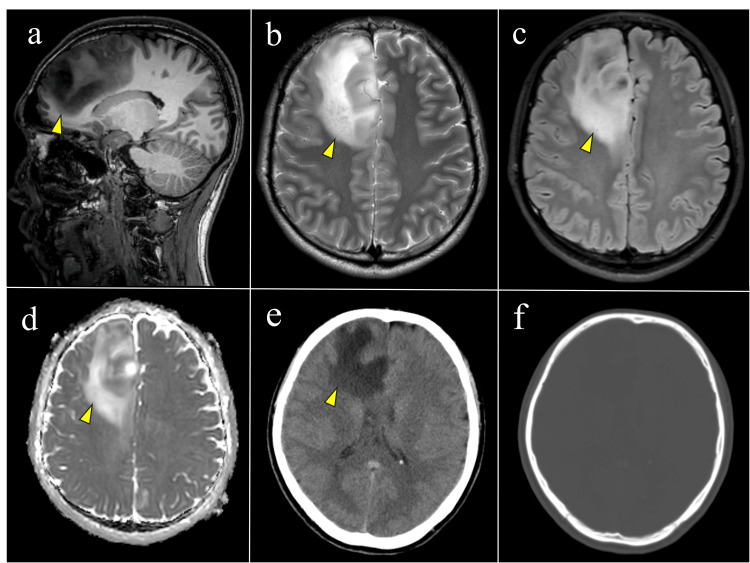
Brain MRI and CT findings (a) Sagittal T1-weighted image showing a hypointense lesion in the deep white matter of the right frontal lobe (yellow arrowhead). (b) Axial T2-weighted image and (c) axial FLAIR image showing a hyperintense lesion with an ill-defined margin. (d) ADC map showing no restricted diffusion in the same region. (e) Non-contrast CT showing a low-density area in the right frontal white matter (yellow arrowhead) without evidence of hemorrhage. (f) Bone-window CT confirming the absence of calcification. MRI, magnetic resonance imaging; FLAIR, fluid-attenuated inversion recovery; ADC, apparent diffusion coefficient; CT, computed tomography
